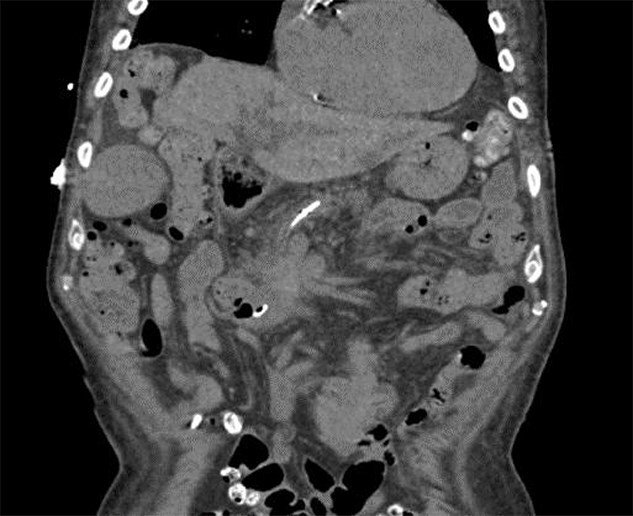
Computed tomography of the abdomen and pelvis demonstrating intact pancreatic duct stent.
